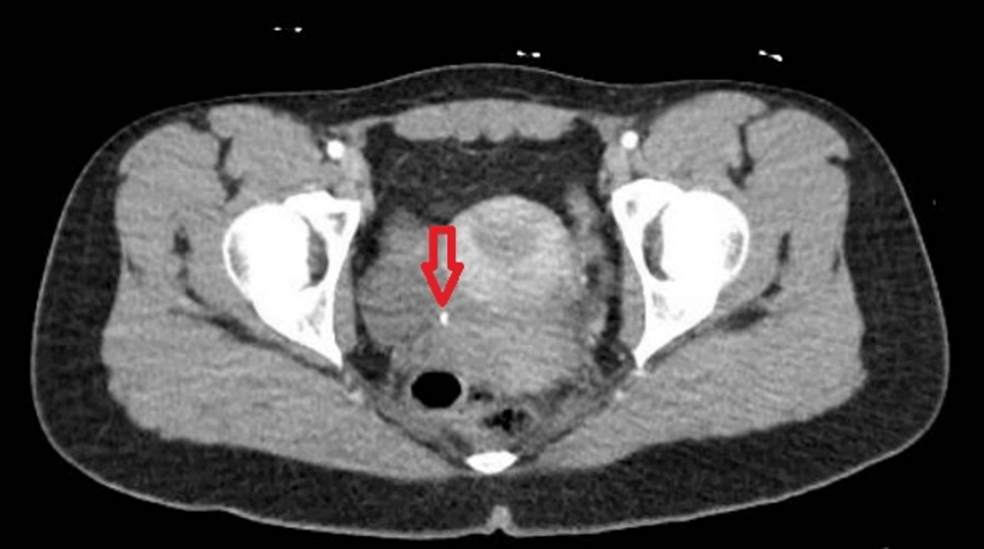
CT abdomen and pelvis showing 3 mm calculus at the ureterovesical junction
